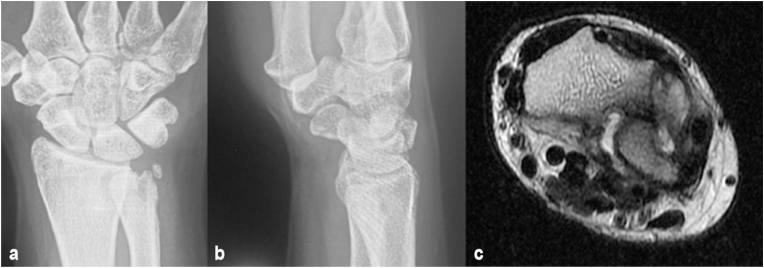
(a,b) Plain X-ray PA and Lateral showing ulnar head fracture and (c) axial MRI showing chronic volar dislocation with impacted fracture of volar-ulnar distal radius.
